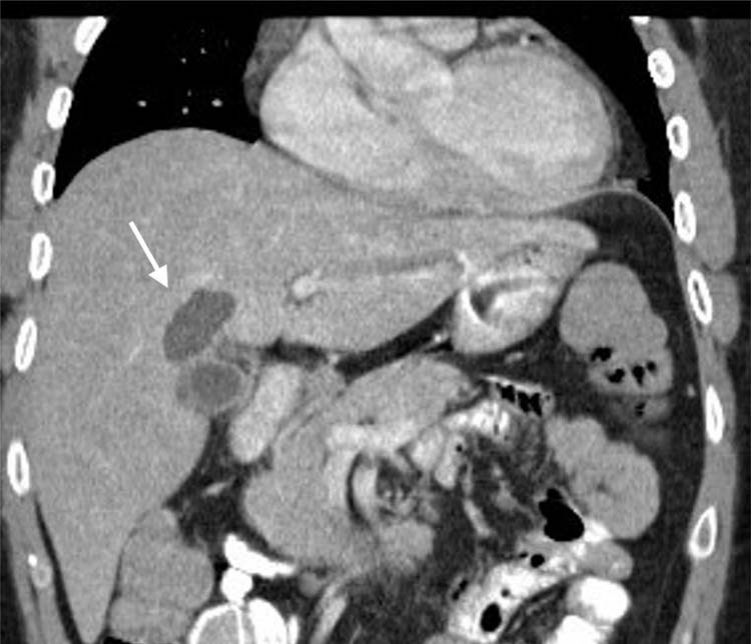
Computed tomography image showing an intrahepatic cyst (arrow).
